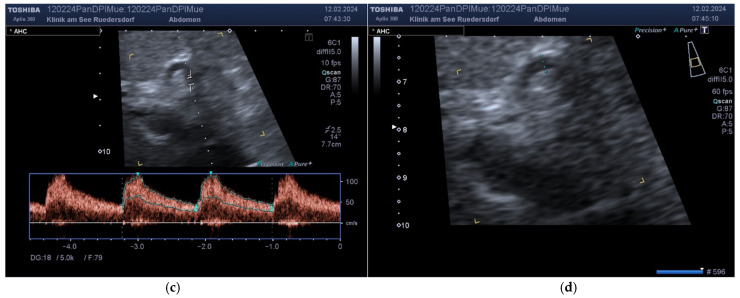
Example of an AHP with a meandering course. (a) Identification with section enlargement. (b) Doppler. (c) Additional Doppler in a further course. (d) Determination of diameter (leading edge method).
